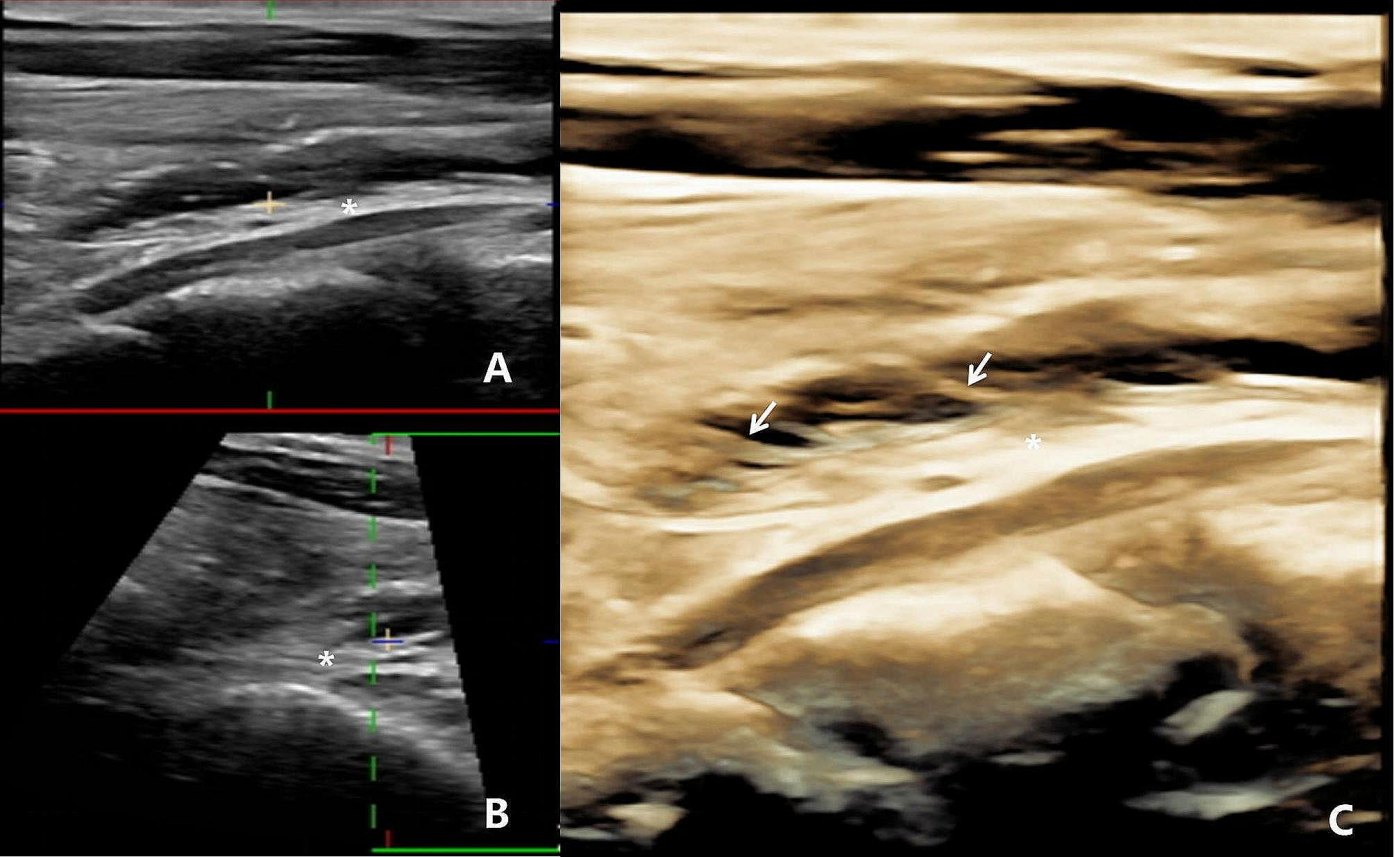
Administration of physiological saline solution between the thyroid capsule and the RLN. (A) Long axial section showing RLN (asterisk); (B) Short axial section showing the RLN (asterisk); (C) Three-dimensional ultrasound imaging showing numerous small branches of the RLN (asterisk) (white arrow)
